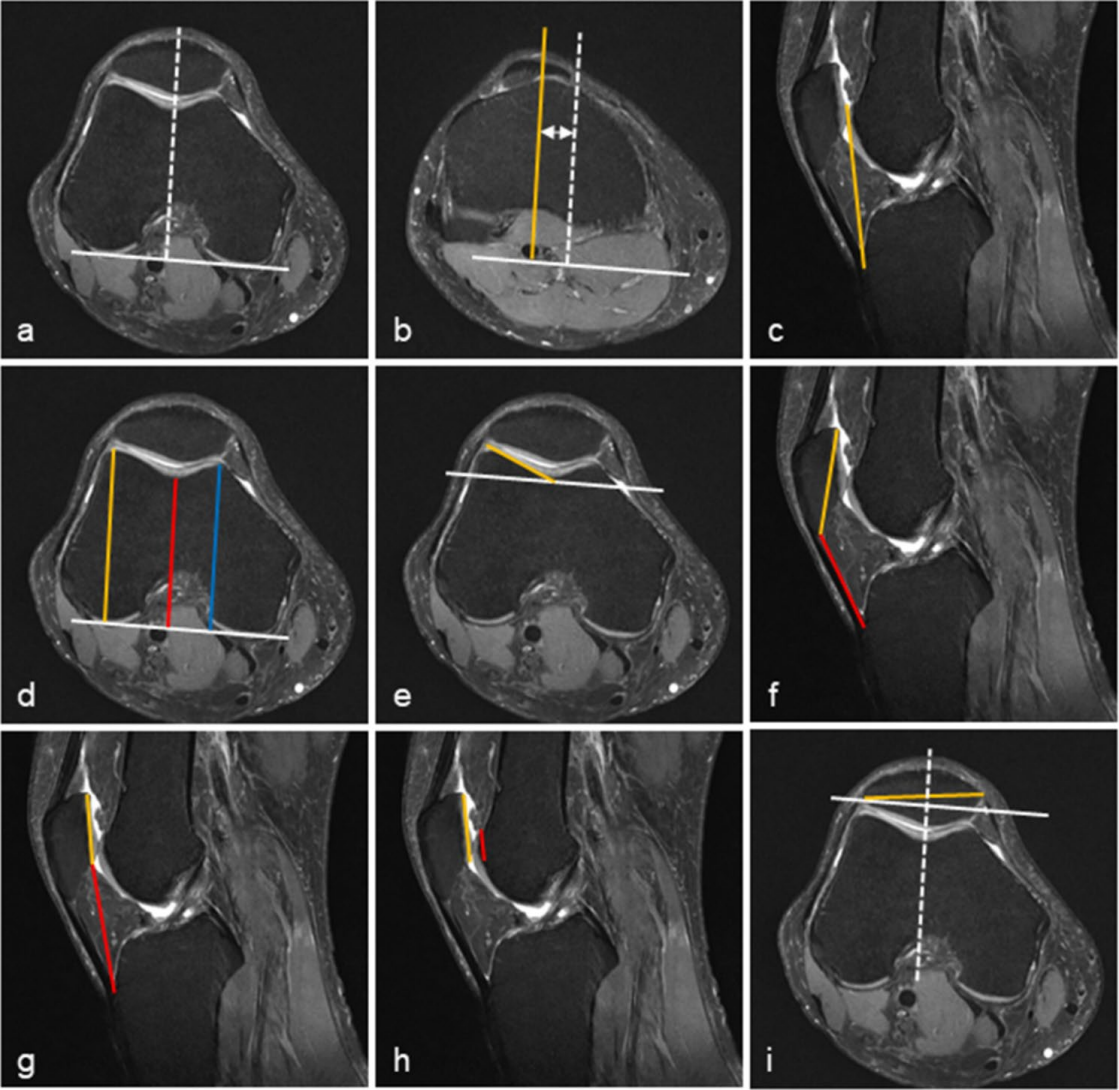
Measurements of the patellofemoral maltracking magnetic resonance imaging parameters. a A baseline (white line) was drawn on the slice where the femoral condyles were at the most posterior. A white dashed line was drawn perpendicular to the baseline, passing through the trochlear groove (TG). b Both lines were transferred inferiorly to the level of patellar tendon attachment. Another perpendicular line (yellow line) was drawn, passing through the center of the patellar tendon attachment (TT). The double arrow shows the TT-TG distance. c The distance (yellow line) between the TG entrance (TE) and patellar tendon attachment (TT) was TT-TE. TT-TG index=(TT–TG)/(TT–TE). d Three lines were drawn perpendicular to the baseline: one from the deepest point of the TG (red line) to the baseline (white line), the others from the medial (blue line) and lateral (yellow line) condyles’ anterior endpoints to the baseline. Trochlear depth was measured by subtracting perpendicular distances of the TG (red line) from the average (blue line and yellow line). e Lateral trochlear inclination was the angle between the baseline (white line) and the line parallel to the lateral trochlear facet subchondral bone (yellow line). f The Insall-Salvati ratio (ISR) was the ratio between patellar tendon length (red line) and patellar height (yellow line). g The modified ISR was the ratio between the distance (red line) from the distal end of the patellar cartilage to the TT and the length (yellow line) of the patellar cartilage. h The patellotrochlear index (PTI) was the ratio between the vertical patellar cartilage distance (yellow line) and the distance (red line) of trochlear cartilage in contact with the patella. i Patellar tilt angle was the angle between the patellar width line (yellow line) and baseline (white line). Bisect offset was defined as the percentage of patella width (yellow line) lateral to midline (white dashed line)
